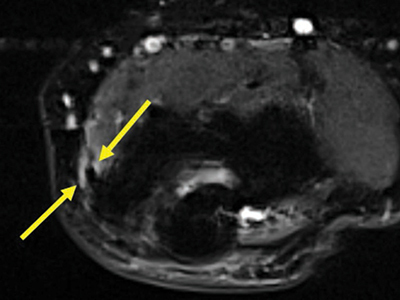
Axial fat-saturated T2-weighted image through the medial flexor origin with a peritendinous T2-hyperintense signal compared to muscle, score 1.
